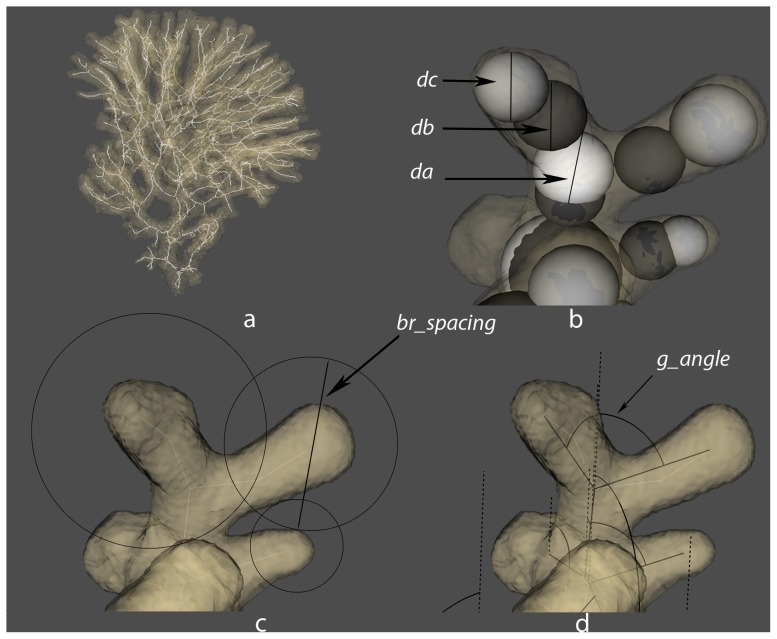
Morphometrics of branching coral colonies. a) Morphological skeleton generated from a volume of a CT scan of a Madracis colony, b) branch thickness, da – white sphere, db – black sphere, dc – gray sphere, c) branch spacing (br-spacing) and d) branching angle relative to the growth direction (g_angle).
